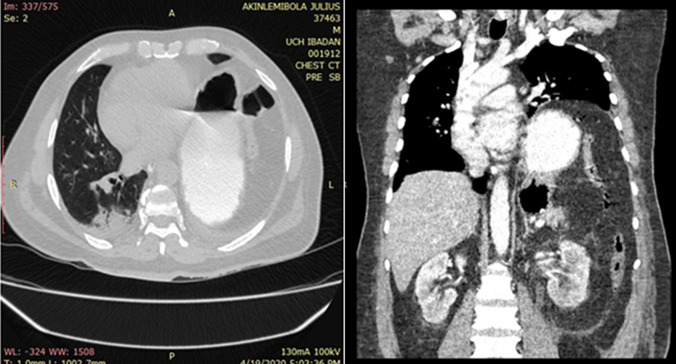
scanogram and axial views of the chest CT scan showing diffuse bilateral peribronchovascular ground glass densities and focal lung consolidation (red arrows) and a mild left pleural effusion (blue arrow)
